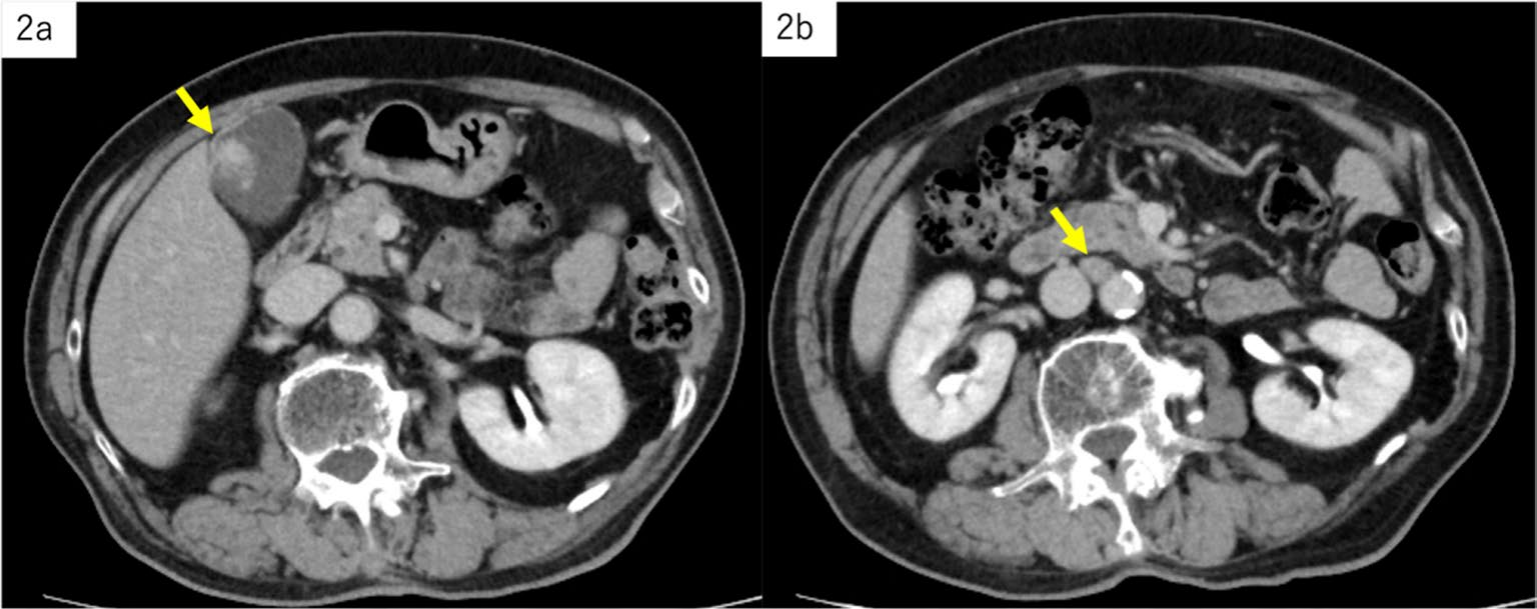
Computed tomography. (a) An inhomogeneous contrast‐affected nodule inside the gallbladder. (b) A 12 mm mass on the right side of the aorta.
